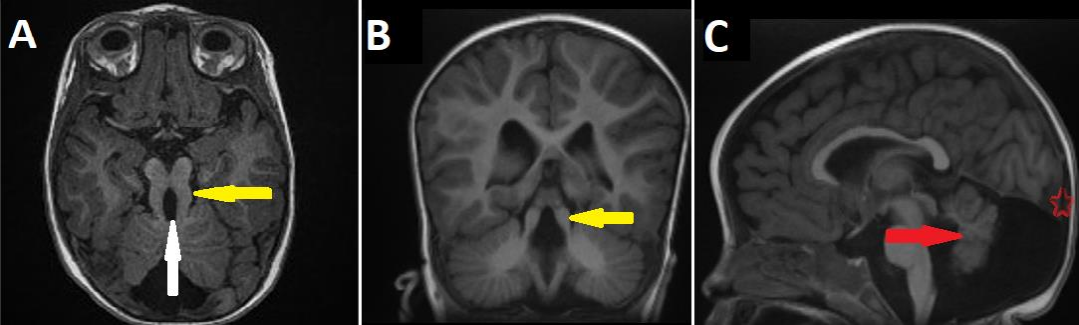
MRI of Joubert syndrome associated with Dandy-Walker malformation; axial plane (A), coronal plane (B), and sagittal plane (C)
